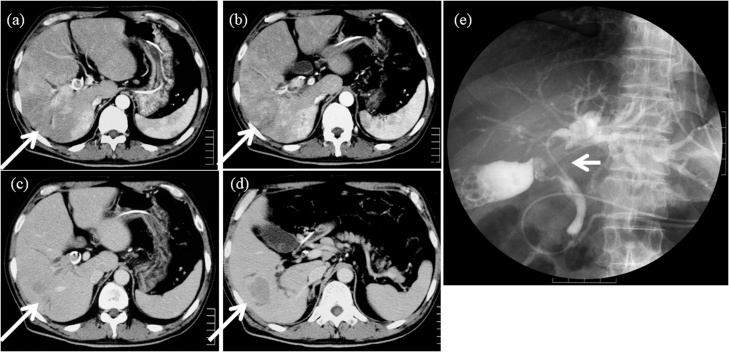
Computed tomography showed a tumor, 40 × 38 mm in diameter, enhanced in the arterial phase (a, b) and washed out in the portal phase (c, d) in segment 6 of the liver (arrow). The right intrahepatic bile duct was dilated and tumor thrombus in the bile duct was detected (arrow head c). Endoscopic retrograde cholangiography showed a filling defect from the right hepatic duct to the common hepatic duct (arrow, e).
